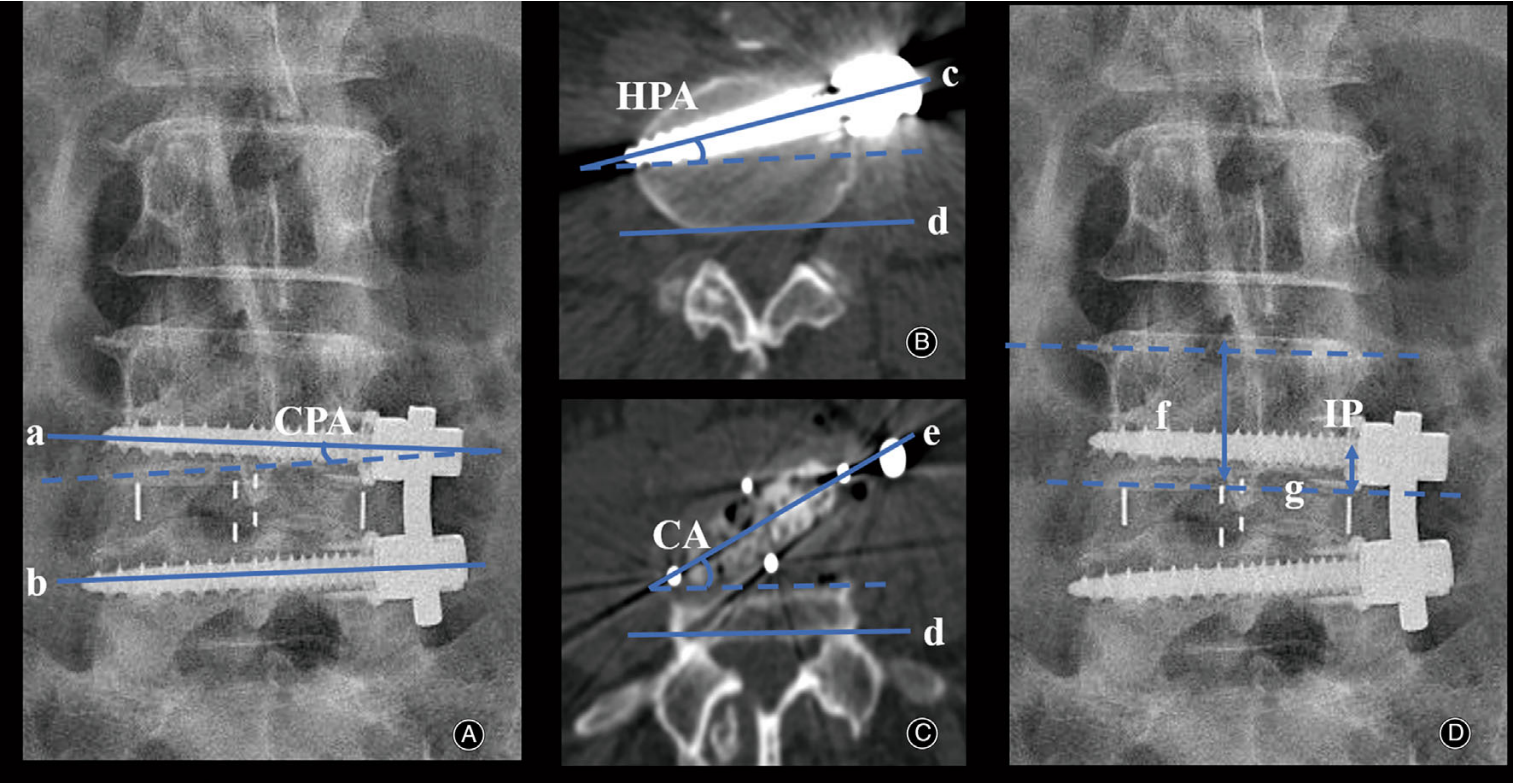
Measurements of radiological parameters related to the vertebral screw measurements. (a) The long axis of the upper vertebral screw; (b) the long axis of the lower vertebral screw; (c) the long axis of the screw; (d) the posterior edge of the vertebral body; (e) the long axis of the cage; (f) the height of the entire vertebral body; (g) the distance between the contact point and the endplate. (A) CPA measured by antero‐posterior X‐ray; (B) CA measured by using axial CT; (C) SA measured by using axial CT; (D) IP measured by antero‐posterior X‐ray.
